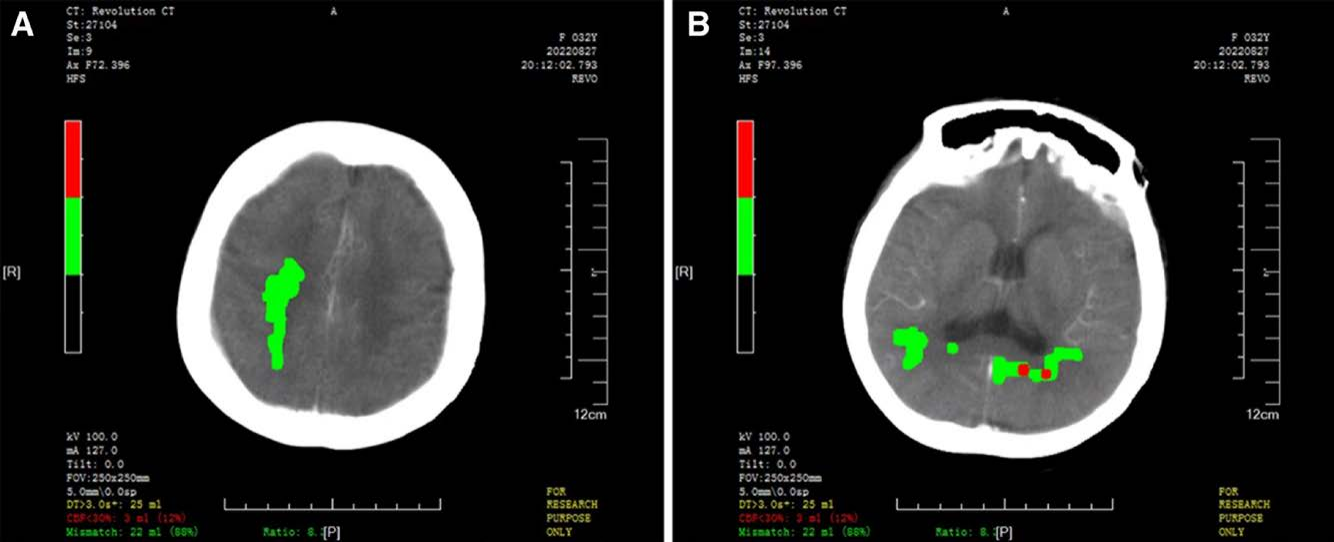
CT perfusion in the acute phase. (A, B) CTP showed a volume mismatch of up to 22 mL between the ischemic core and hypoperfusion area. CT = computed tomography, CTP = CT perfusion.
